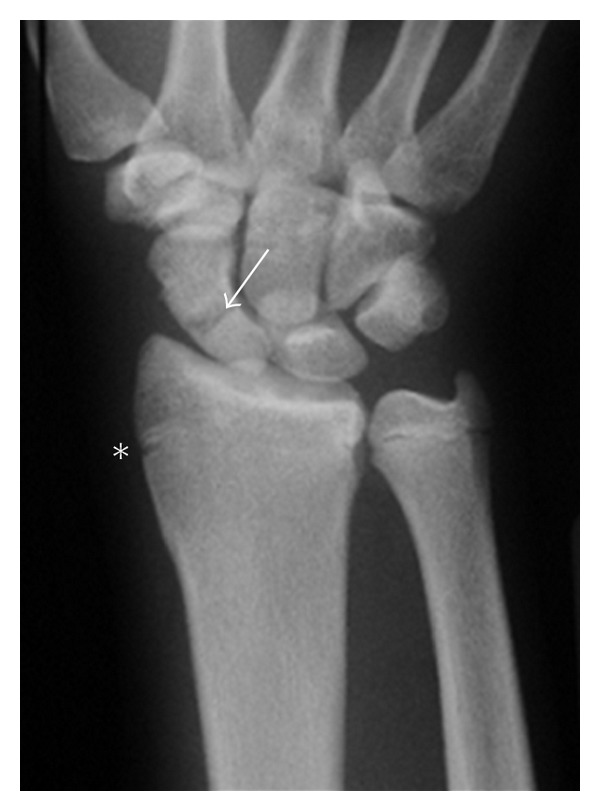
Plain coronal radiograph suggesting fracture at the waist of the scaphoid (arrow) and widening of the radial aspect of the distal radial epiphysis (∗).
